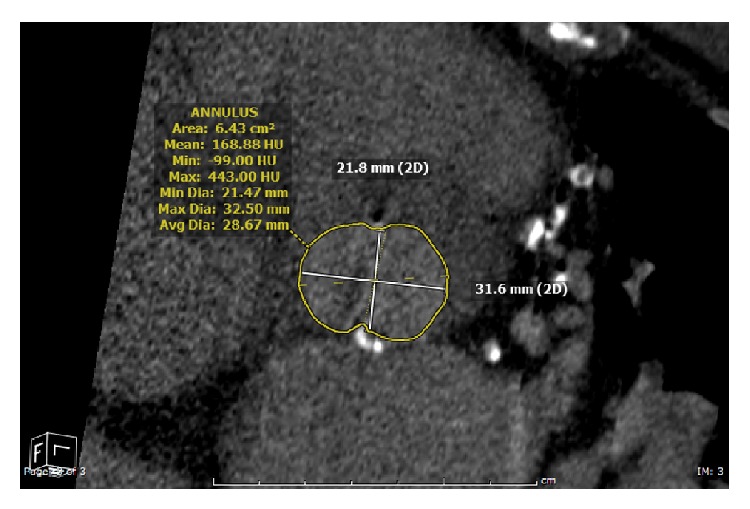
CT determined cross-sectional aortic annular dimensions.
